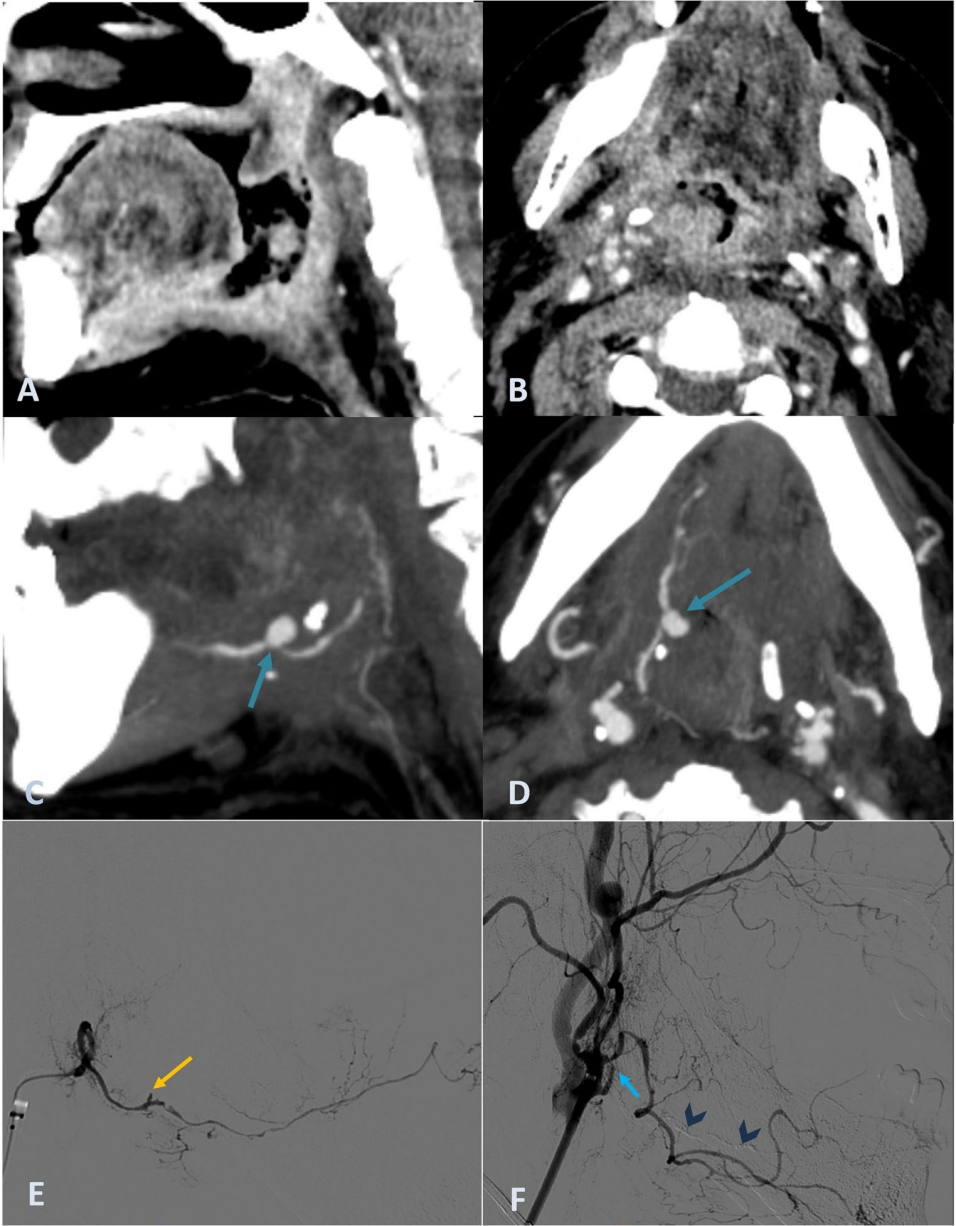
CE-CT images in sagittal (A) and axial (B) planes showing an infiltrative lesion involving the neopharynx walls, with extension into the right tonsillar region and tongue base, consistent with recurrent tumour. CTA in sagittal (C) and axial (D) planes demonstrating a pseudoaneurysm of the right lingual artery at the tongue base (*blue arrows*), located beneath the area of tumour ulceration. DSA identified the rupture site of the right lingual artery (E)
